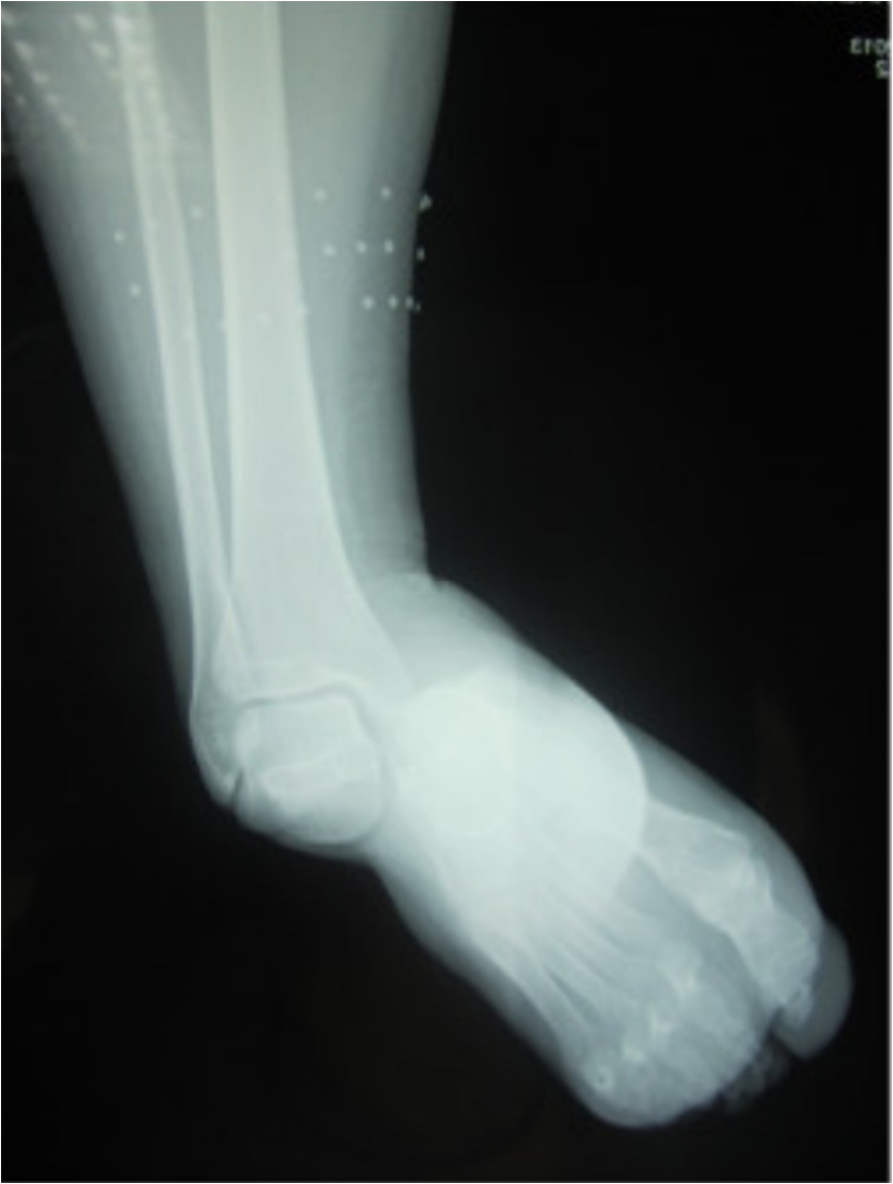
Anteroposterior radiograph of the ankle showing medial subtalar dislocation without fractures.
